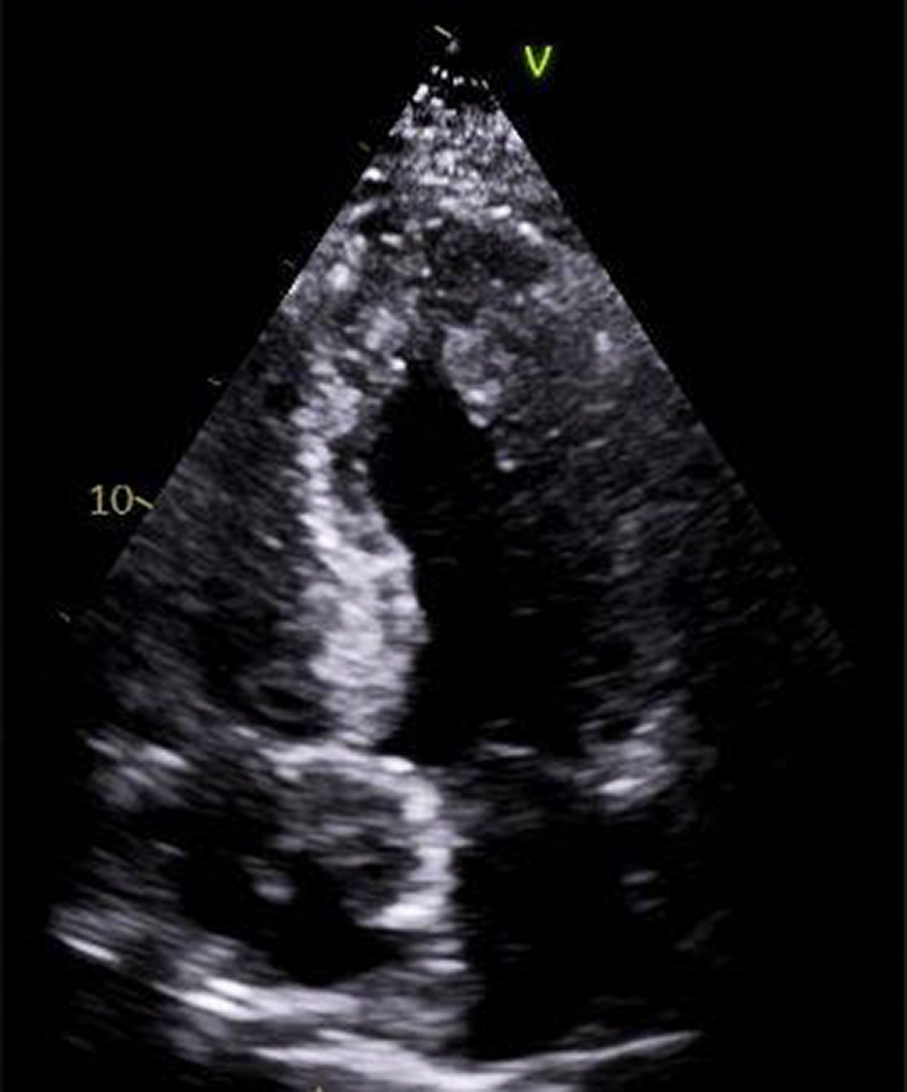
Apical 4 chamber view showing asymmetric left ventricular hypertrophy with abnormal appearance of myocardium
